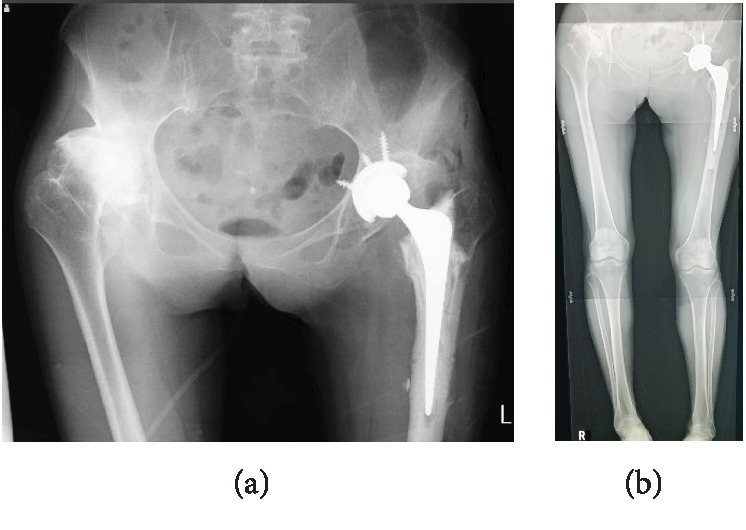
Postoperative plain radiograph. Hybrid THA was performed. (a) Anteroposterior view; (b) whole lower extremities in standing position.
